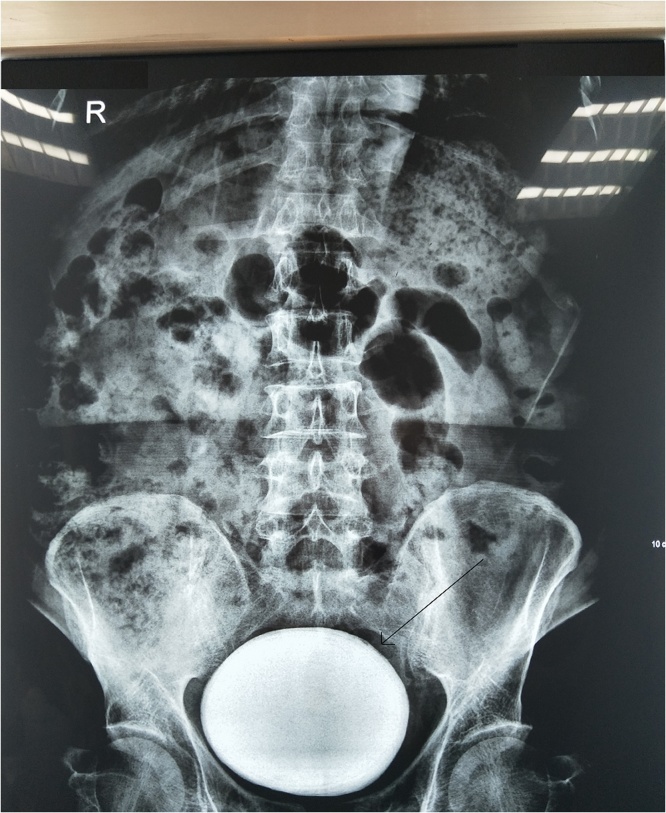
X-ray film showing giant, regular bladder stone.
